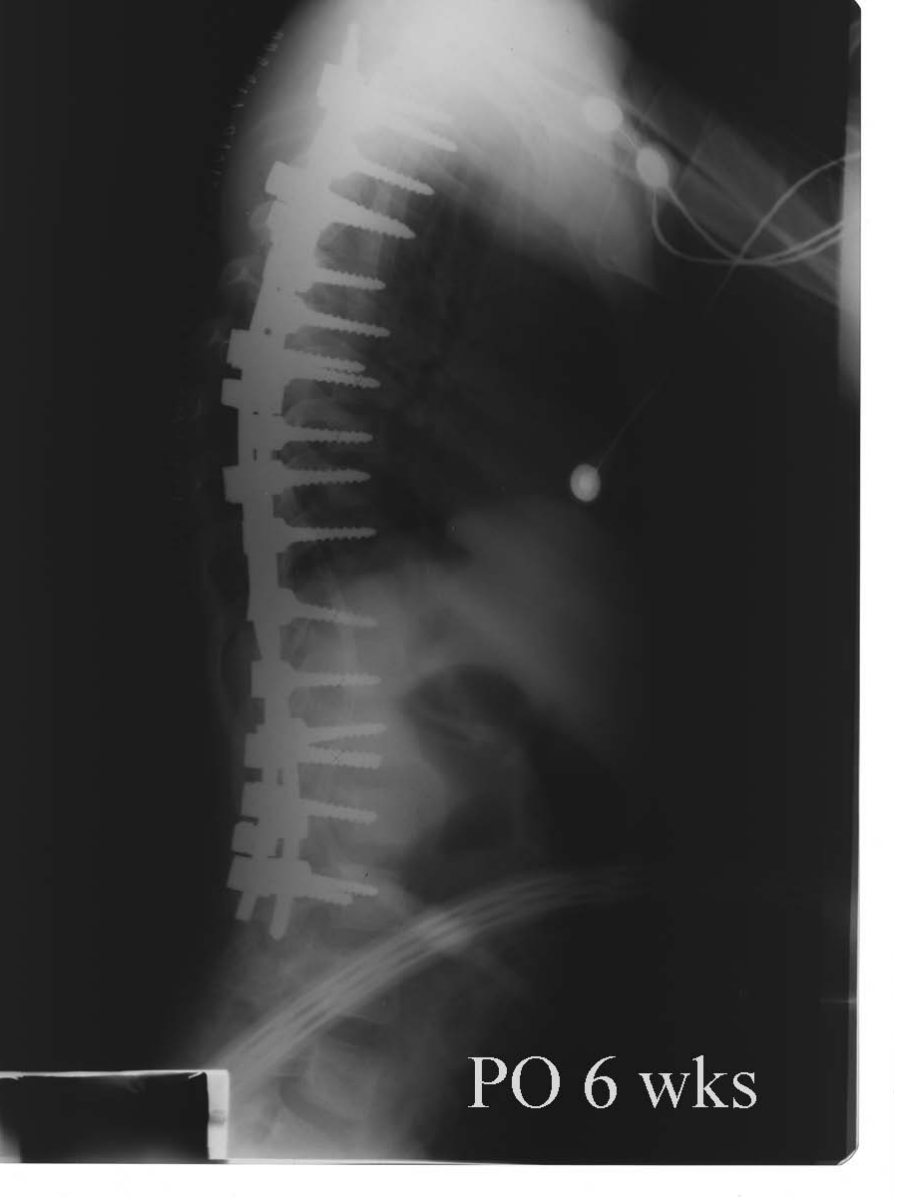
Postoperative lateral radiograph of the same patient taken 6 weeks after surgery. Thoracic kyphosis and lumbar lordosis angles were 44.9° and 35.5°, respectively.
